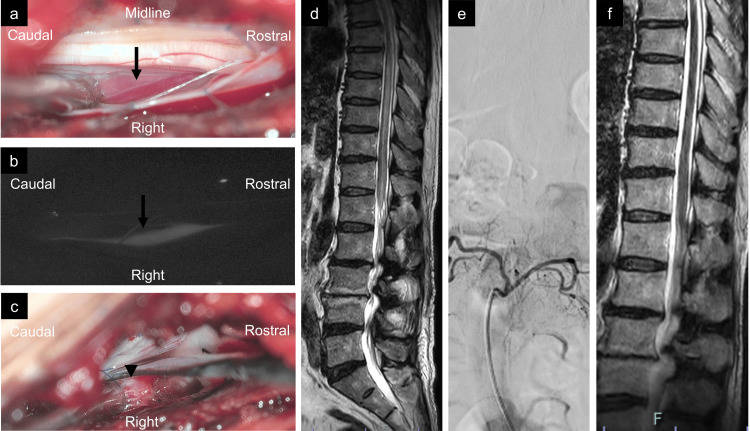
Intraoperative and postoperative radiological findings. (a,b) After opening the dura, a dilated reddish vein (black arrow) was identified. Early retrograde flow in the vein was observed with ICG (b). The veins were coagulated and obliterated. (c) The shunting pouch (black arrowhead) of the epidural arteriovenous fistula was ventrally identified after dural retraction. (d) Postoperative sagittal T2-weighted MRI showing disappearance of flow voids but with residual edema of the spinal cord. (e) Postoperative digital subtraction angiography revealing the disappearance of the epidural arteriovenous fistula. (f) Sagittal T2-weighted MRI four months after surgery showing improvement in spinal cord edema. ICG: indocyanine green; MRI: magnetic resonance image
